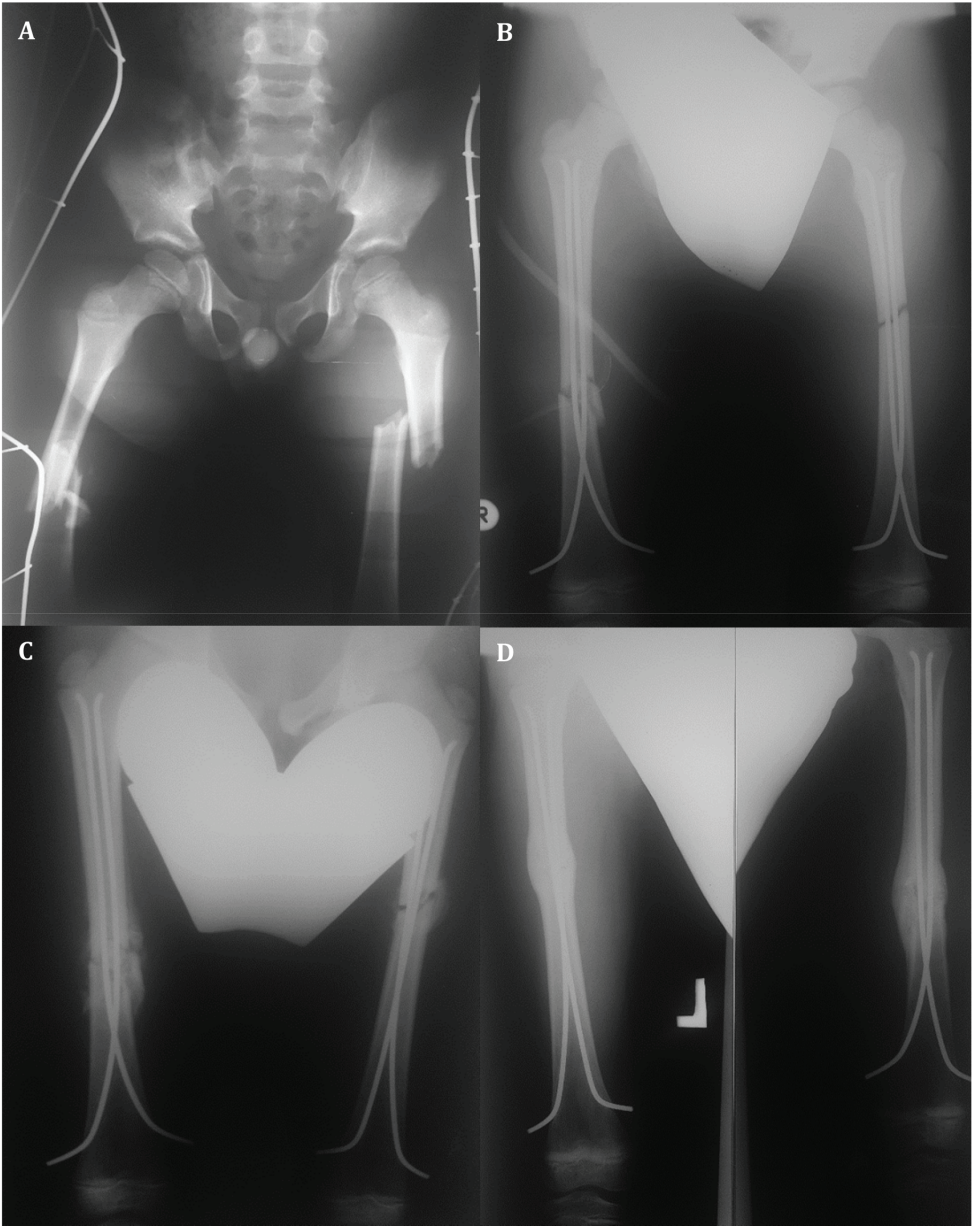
A 8-year-old boy with bilateral diaphyseal femoral fracture: A. Preoperative radiography; B. Intraoperative radiography; C. One month after surgery; D. Three months after surgery – complete healing of the fracture
